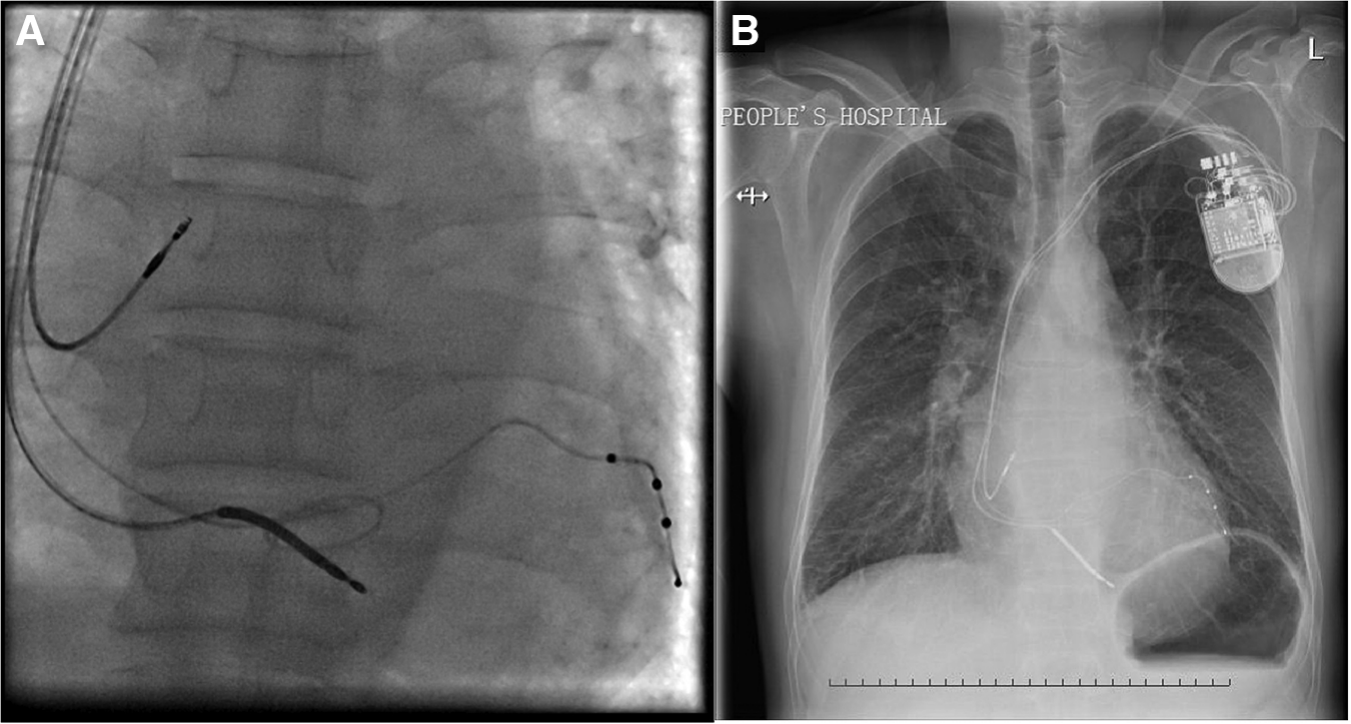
(A) Final position of the left ventricular lead by the loop technique from right anterior oblique projection. (B) Chest x-ray image of the position of the left ventricular lead by the loop technique at the 3-year follow-up.
